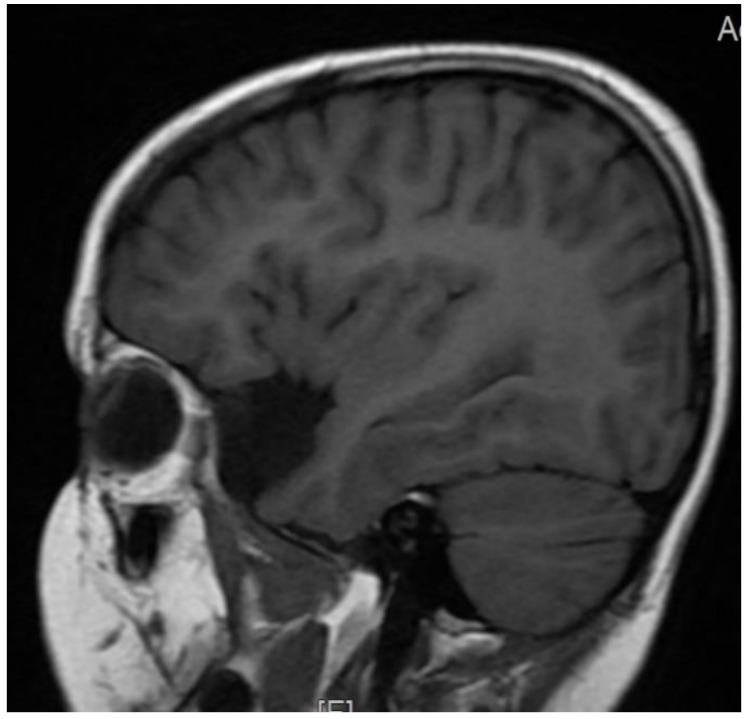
T1-weighted sequence. Cyst modeling temporal lobe.
